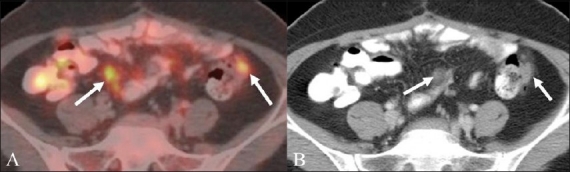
A 39-year-old woman with a history of CRC. Axial fusion PET / CT (A) image shows hypermetabolic FDG-avid nodular foci abutting the bowel surface. Axial contrast-enhanced CT scan at the same anatomical level (B) shows enhancing nodular serosal deposits along the bowel surface (arrows) corresponding to the hypermetabolic foci in (A). Note the striking conspicuity of the deposits in (A) as compared to the subtle findings in (B)
